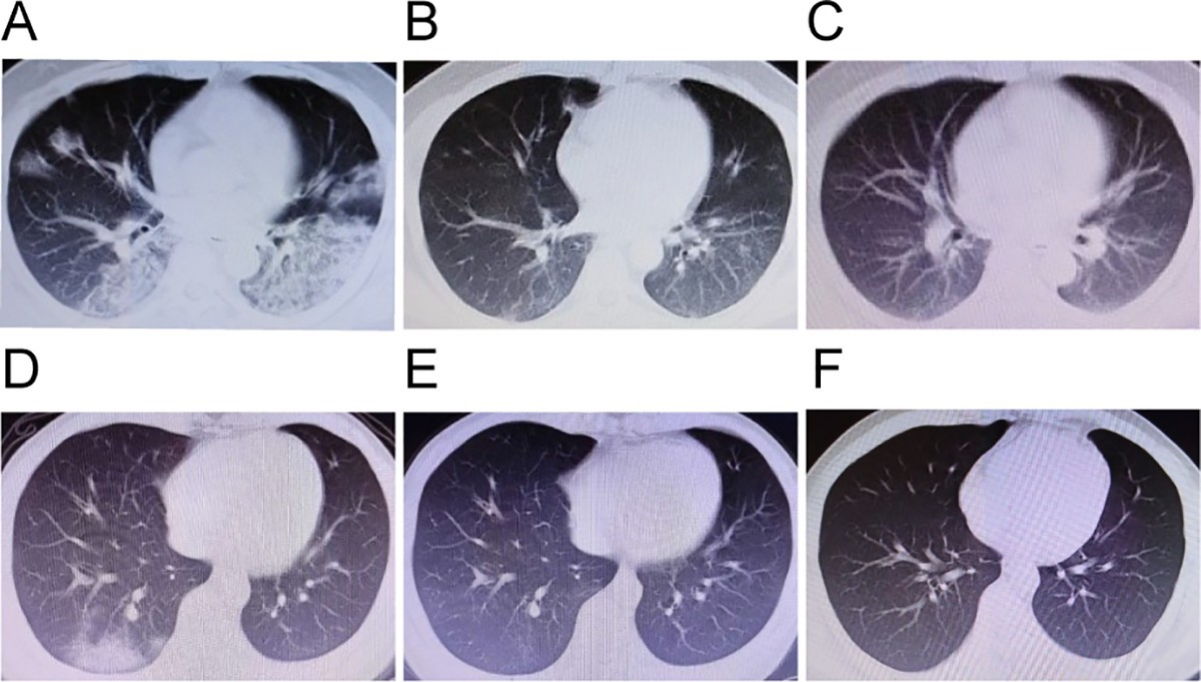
Chest CT images. The representative chest CT images of the moderate survivors. (A-C) A 38-year-old man showed bilateral ground-glass opacity co-existed with subsegmental areas of consolidation on admission (A), the above lesion improved significantly at discharge (B), and the above lesion improved further 4 weeks after discharge (C). (D-F) A 26-year-old man showed bilateral ground-glass opacity on admission (D), the above lesion improved significantly at discharge (E), and the above lesion absorbed completely 4 weeks after discharge (F).
